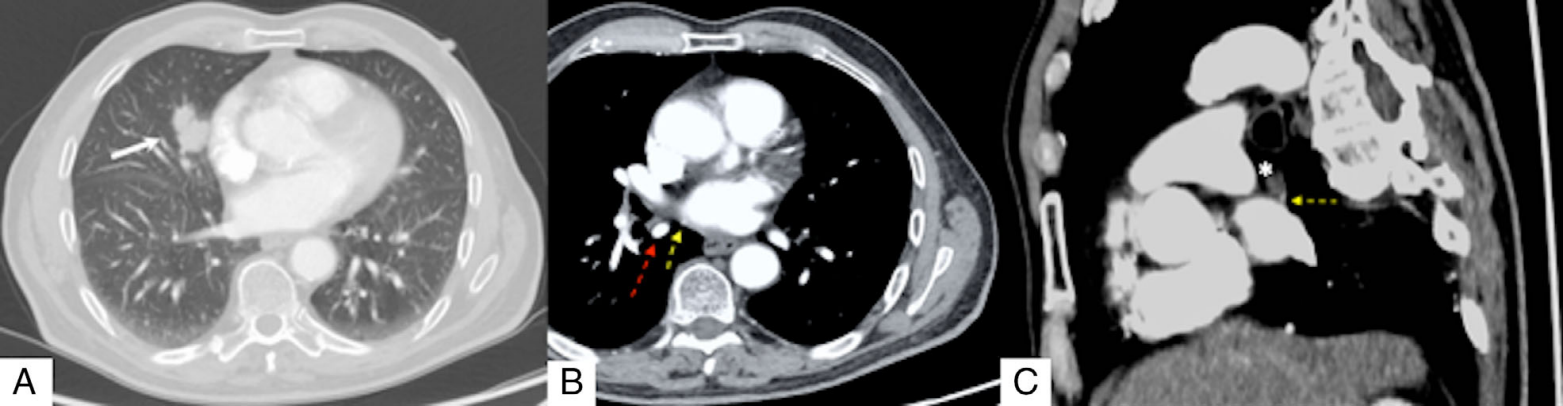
Chest computed tomography (CT) scan. (A) Lung cancer located in the middle lobe (white arrow). (B) Mediastinal window image shows vein (red arrow) and accessory vein (accessory V^6^) (yellow arrow) draining the superior segment of the right lower lobe. (C) Sagittal multiplanar reconstructed (MPR) projection shows accessory V^6^ (yellow arrow) adjacent to mediastinal lymphadenopathy (white asterisk).
